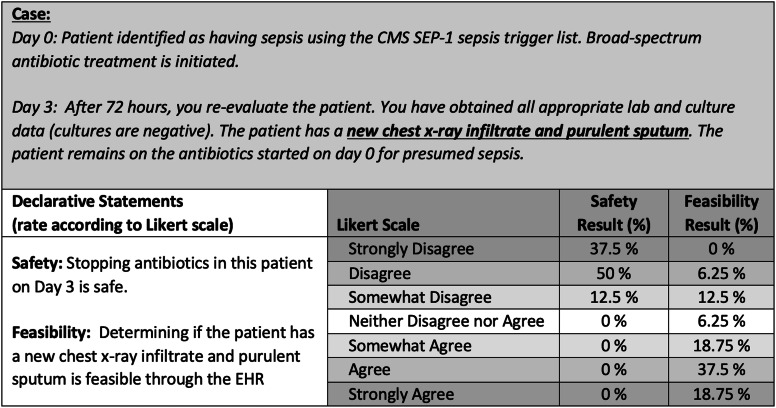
Case-scenario and safety and feasibility declarative statements example. Note. Each criterion was discussed during round 3 due to the survey results indicating <80% agreement in the feasibility of this parameter being identified by chart review. The expert panel agreed to modify and combine criteria to exclude patients with new chest radiograph infiltrate with or without purulent sputum.
